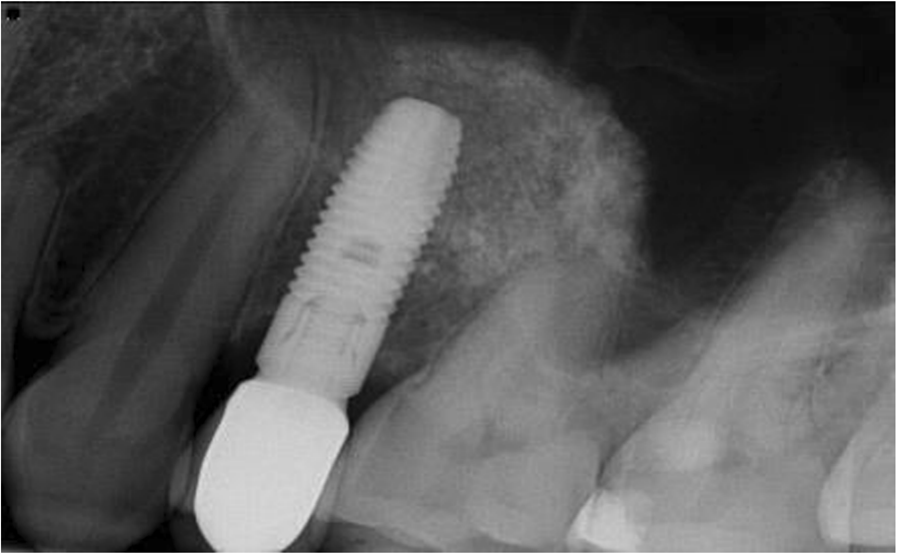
Periapical radiograph of a standard length implant in conjunction with MSFA one-year after functional loading
